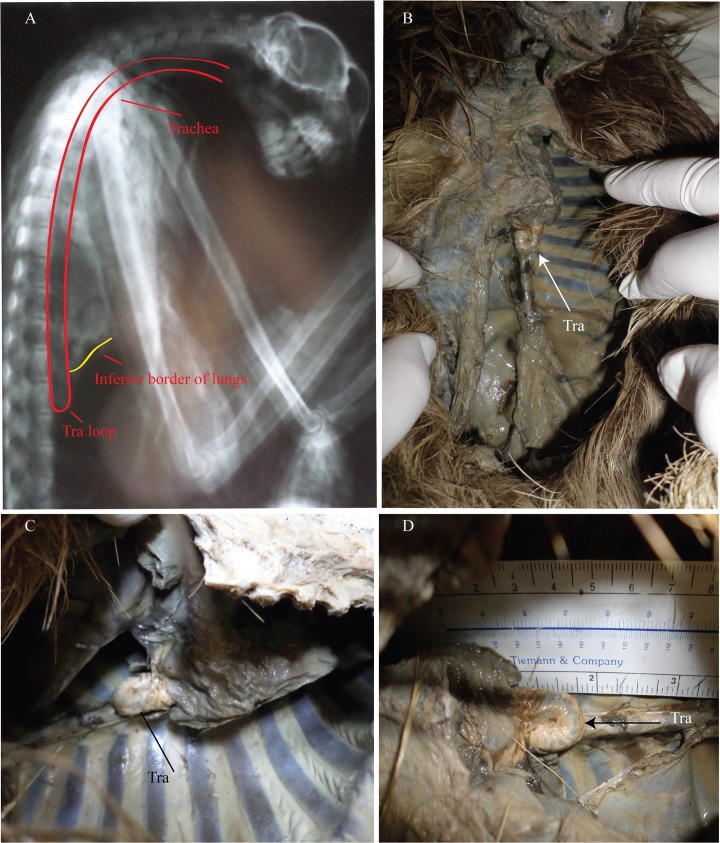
Bradypus tridactylus* views of the tracheal loop. (A) lateral radiograph of the course of the trachea. (B-D) views of the tracheal loop. Tra = trachea; Tra loop = tracheal loop.
